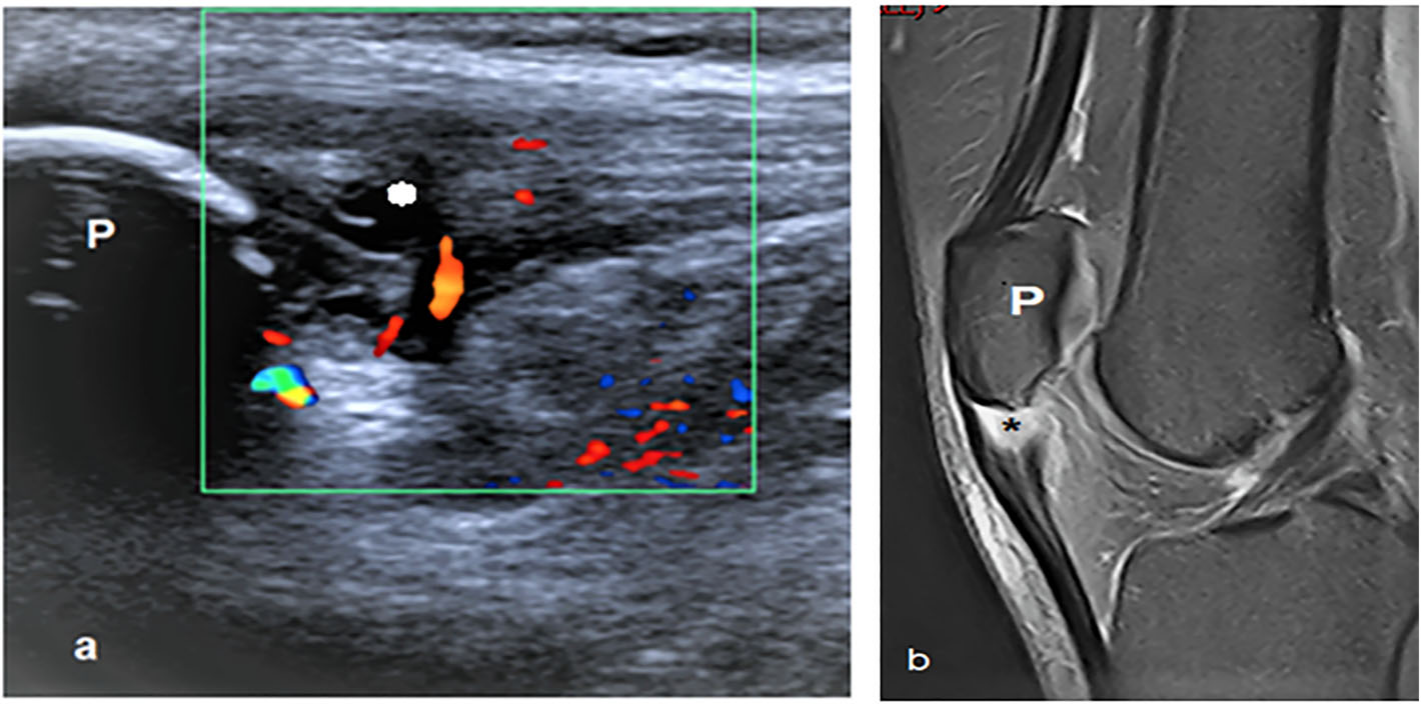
A 22-year-old male with left patellar tendinopathy. a Long axis ultrasound image demonstrates the thickened proximal part of the patellar tendon with cystic changes (*). Color Doppler mapping shows increased vascularity in and around the tendon. b Sagittal PDFS shows thickened proximal patellar tendon with fluid signal (*). P, patella
